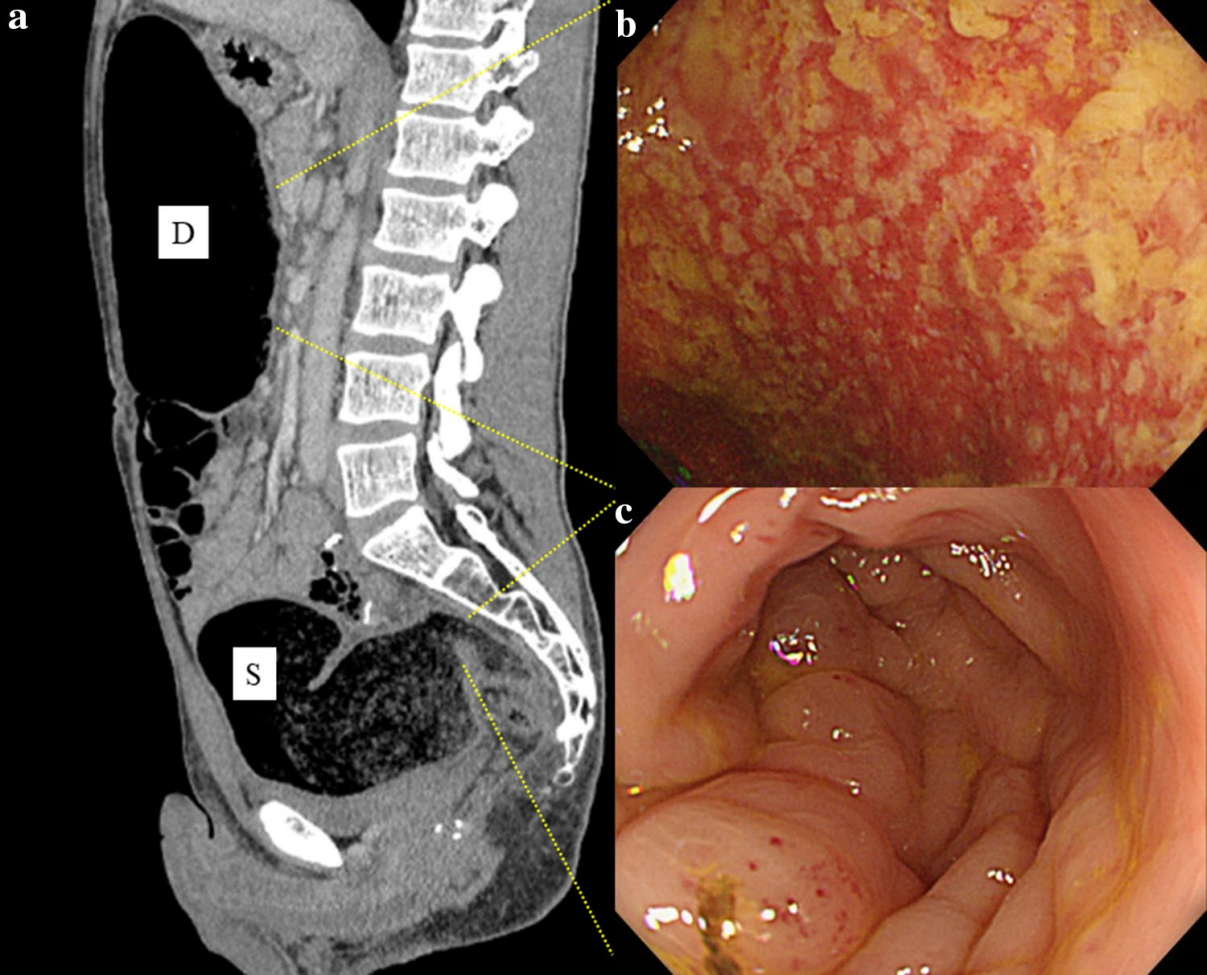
Abdominal CT and colonoscopy findings following stoma closure. CT showed a marked dilatation from the ascending to sigmoid colon (a). Colonoscopy revealed a mucosal erosion and pseudo-membrane in the descending colon (b). Colonoscopy also revealed an edematous mucosa in the rectosigmoid colon (c). Pseudomembranous enterocolitis or cytomegalovirus-induced enterocolitis were suspected
